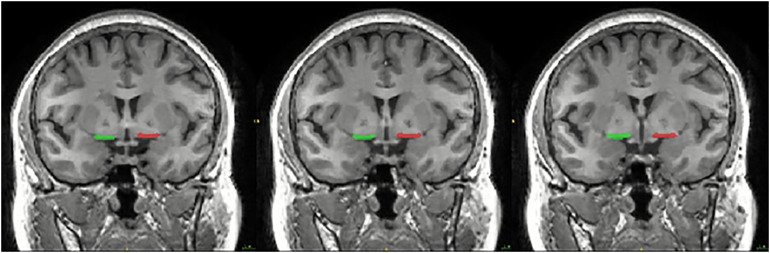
Coronal slice of the MRI image showing the location of the substantia innominate (SI) used in the seed-based resting-state functional connectivity (rsFC) analysis. The SI was drawn at three consecutive gapless 1.2 mm-thick slices on T1-weighted coronal images reformatted to be perpendicular to the anterior commissure (AC)-posterior commissure (PC) line. The three consecutive sections analyzed were located at the level of the crossing of the AC, the level where the AC might be uncrossed, and the level of the emergence of the AC from the temporal lobe. The boundaries of the SI were as follows: the dorsal border was the ventral aspect of the globus pallidus, the ventral border was the base of the brain containing the anterior perforated space, the medial border was operationally defined by a vertical line extending from the ventrolateral border of the bed nucleus of the stria terminalis to the base of the brain, and the lateral border extended to the medial aspect of the putamen.
